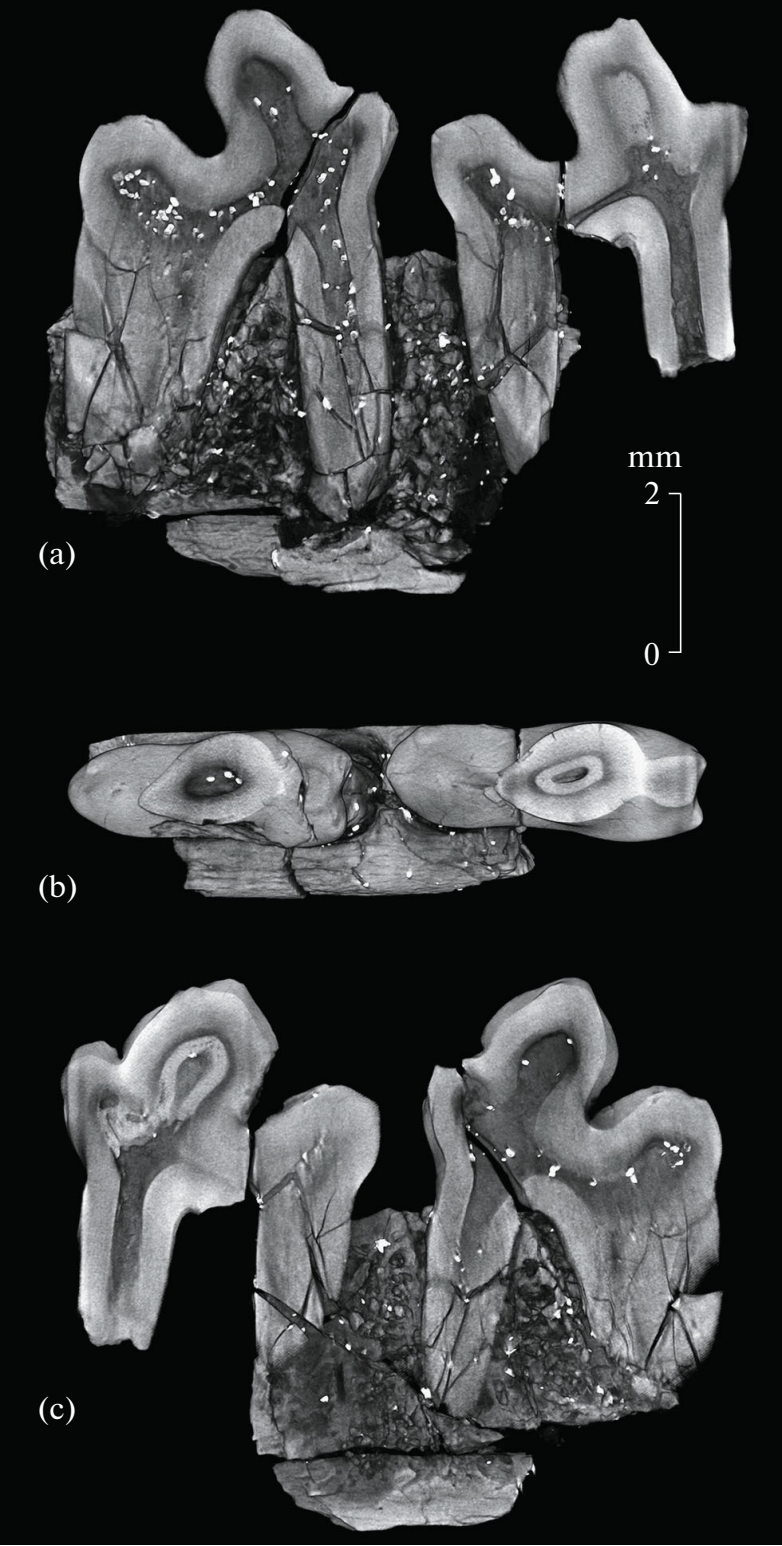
Hapalodectes paradux* Lopatin, sp. nov., holotype PIN, no. 3104/775, right dentary fragment with M_2_–M_3_, CT model: (a) sagittal section, labial view; (b) frontal section ventral to the paraconid apices, occlusal view; (c) sagittal section, lingual view; Mongolia, Tsagan-Khushu; Upper Paleocene, Naran Bulak Formation, Zhigden Member.
